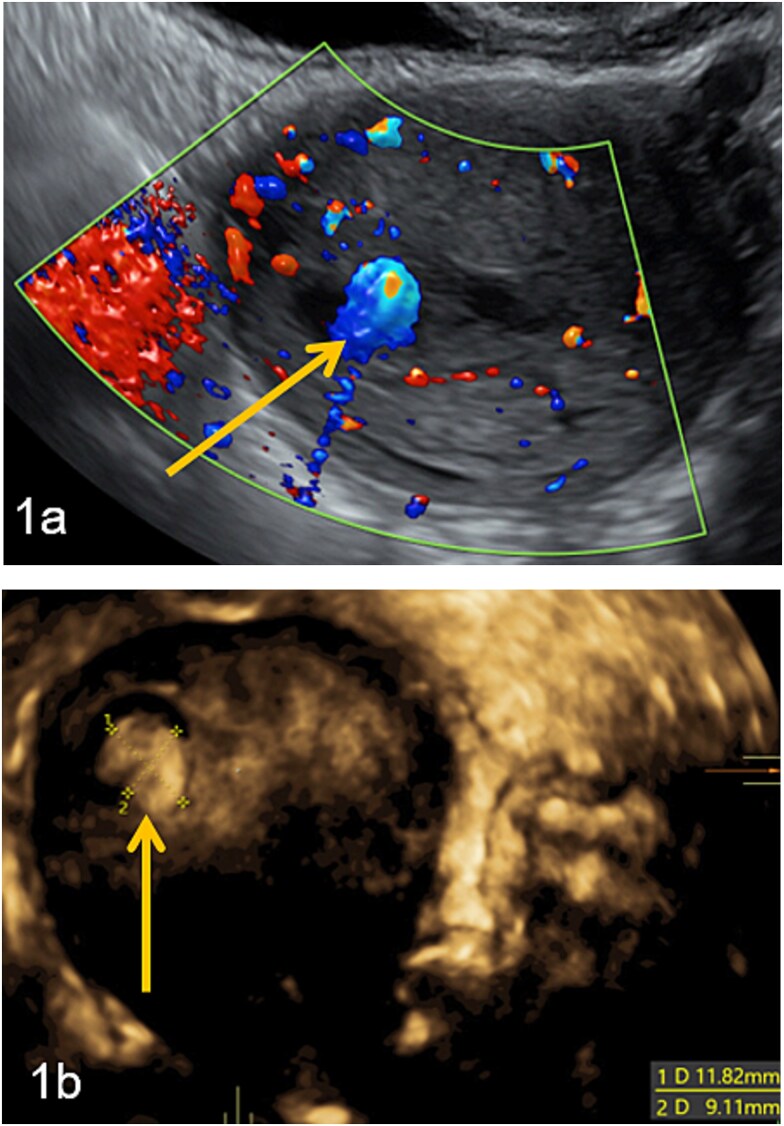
Intra-uterine vascularized mass (arrows) diagnosed by Doppler (a) and 3D-pelvic (b) ultrasound. The Doppler color score is 4 and there is no suspicion of myometrial invasion (continuous halo surrounding the lesion).
